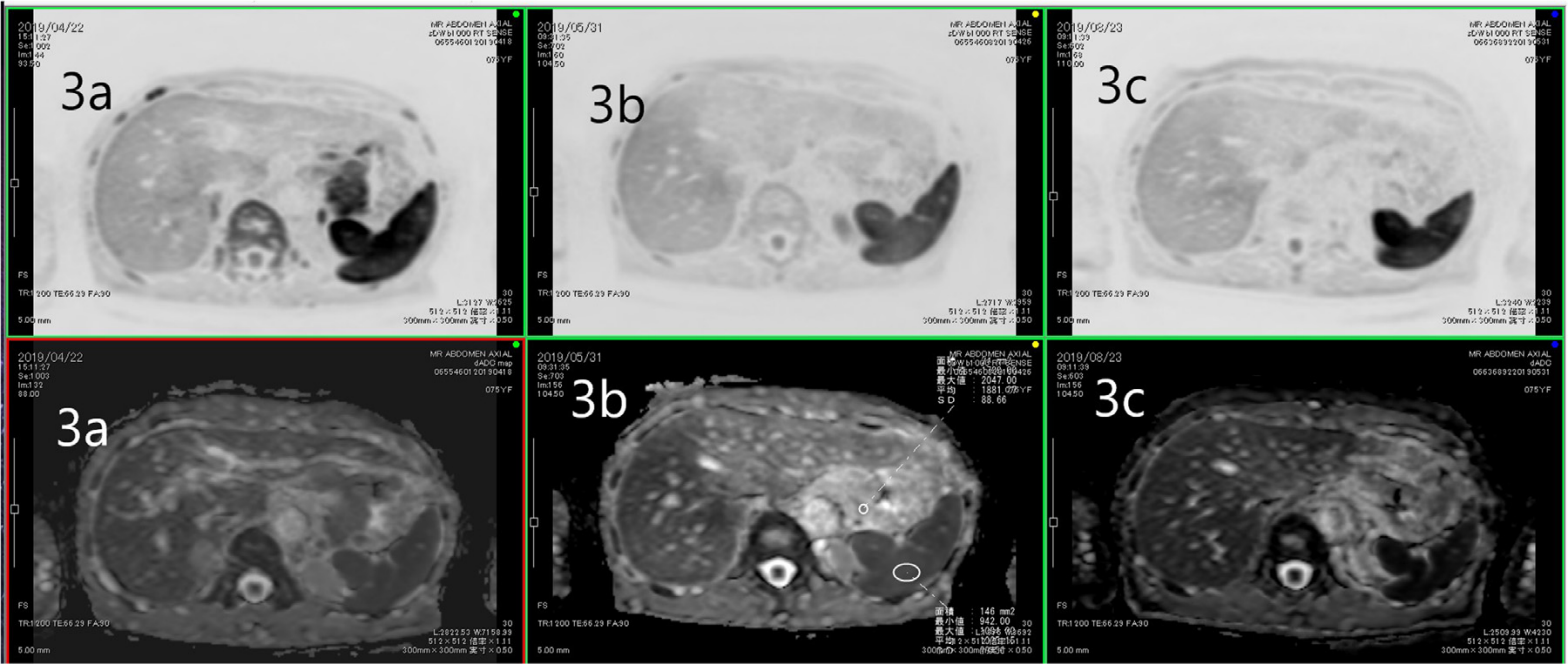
Change in the magnetic resonance (MR) diffusion-weighted imaging (DWI). (A) Day 0 of radiotherapy: High intensity of the increased gastric wall thickness is shown. No obvious change from before radiotherapy in comparison with the spleen's intensity is observed. (B) Diffusion-weighted image 2 months after the initial radiotherapy. The gastric wall signal intensity is decreased relative to that in the previous study. (C) Four months after the initial radiotherapy. The gastric wall signal intensity was the same as that in the previous study
